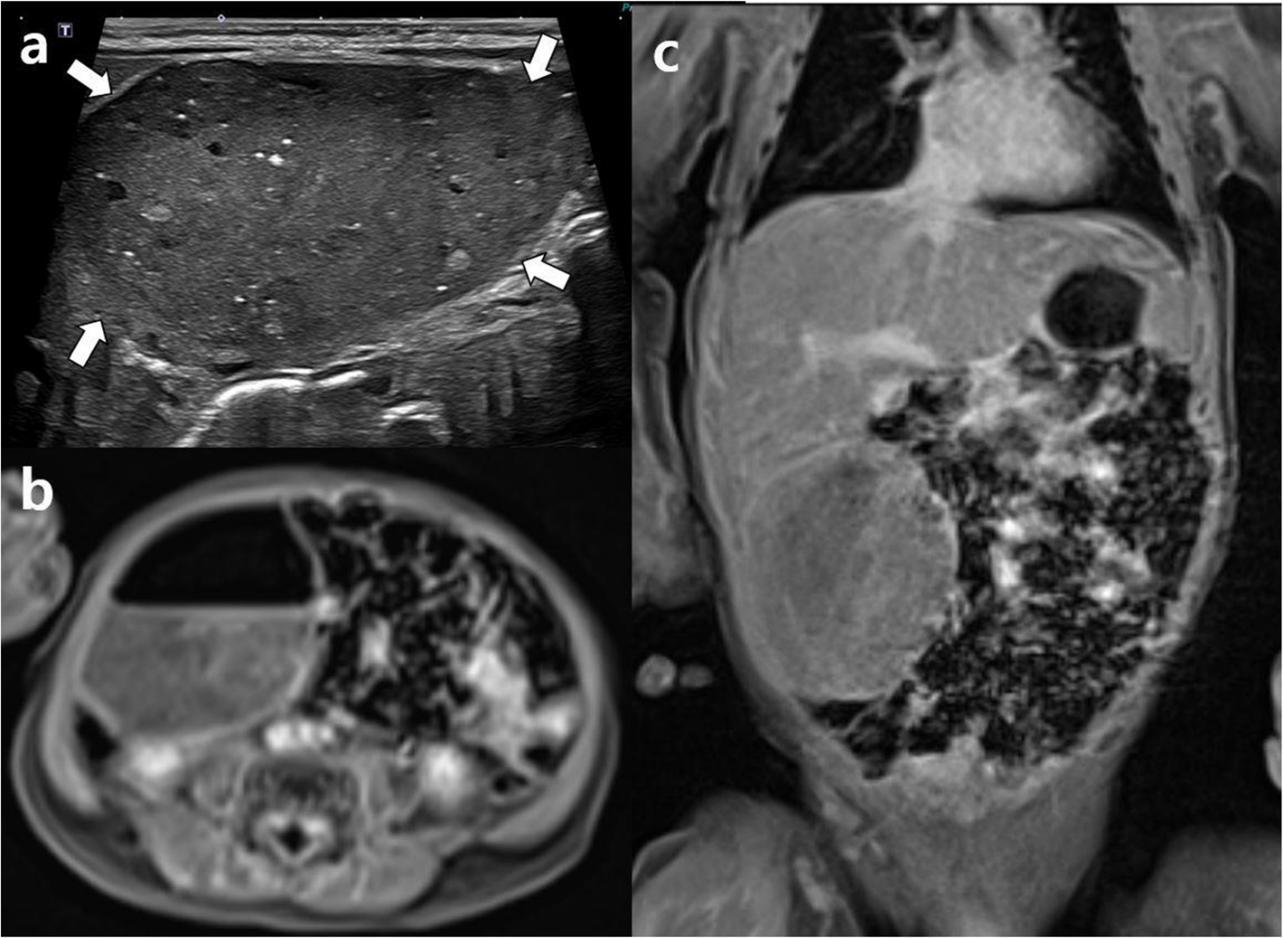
a A transverse sonogram shows a cyst with echogenic material, fluid, and calcification (arrows). It originated from the retroperitoneum and is located in front of the inferior vena cava and descending aorta. The size of the cyst is 4.2 × 2.3 × 4.2 cm. Contrast-enhanced fat-saturated T1-weighted MR image of transverse (b) and coronal (c) view reveal a cystic mass with air-fluid level and multiple tiny low signal intensity component. Size of cystic mass is 4.6 × 4.5 × 5.3 cm
